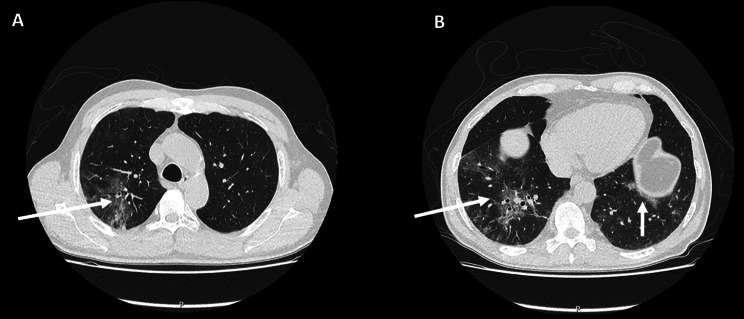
Chest computed tomography acquired on the admission day (3 days before IVIG Infusion). (A) Multifocal ground glass opacities in the right upper lobe (white arrow. (B) Multifocal ground glass opacities in the in the right and left lower lobe (white arrows)
